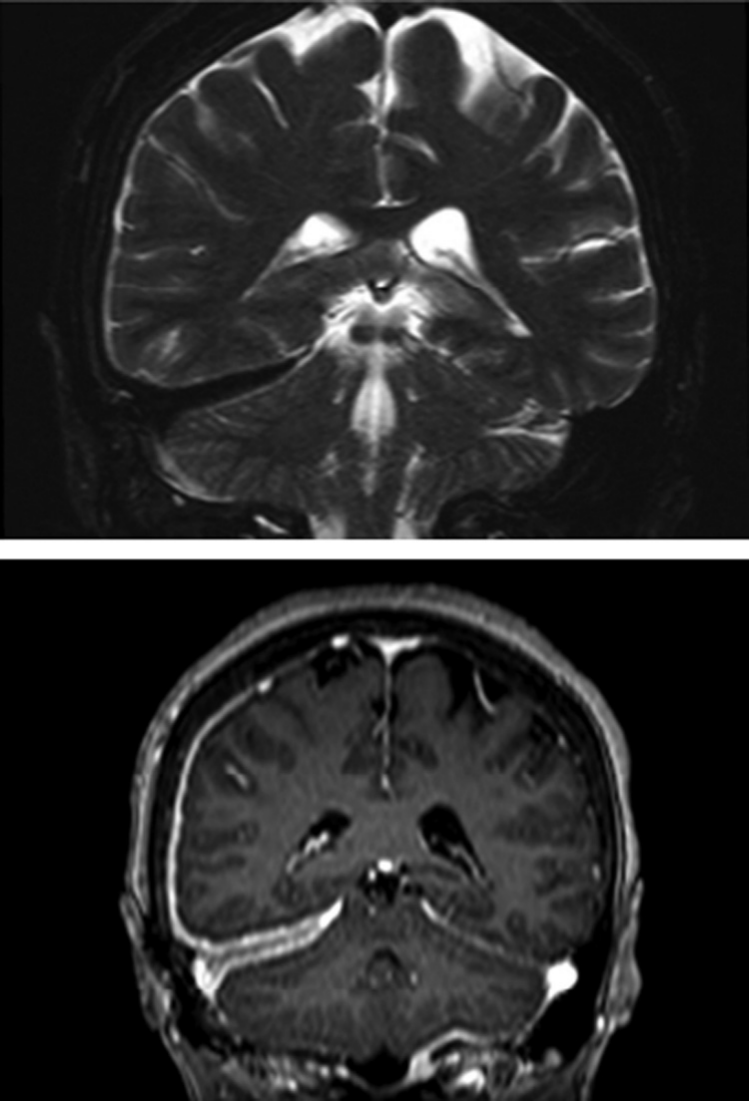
T2 (upper) and postcontrast T1 (bottom) weighted magnetic resonance imaging (MRI) scans, showing dural thickening on coronal view before treatment.
